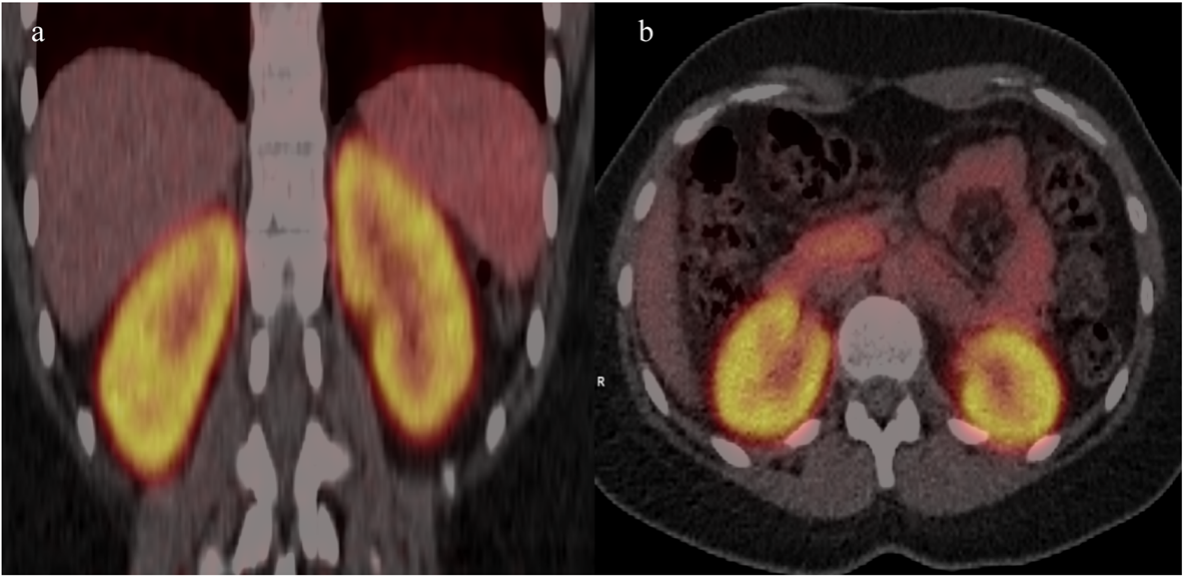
Typical example of a coronal and b transaxial PET/CT images of kidneys during maximal ^82^Rb uptake from one of the study subjects. Shown example is for FOV-B
